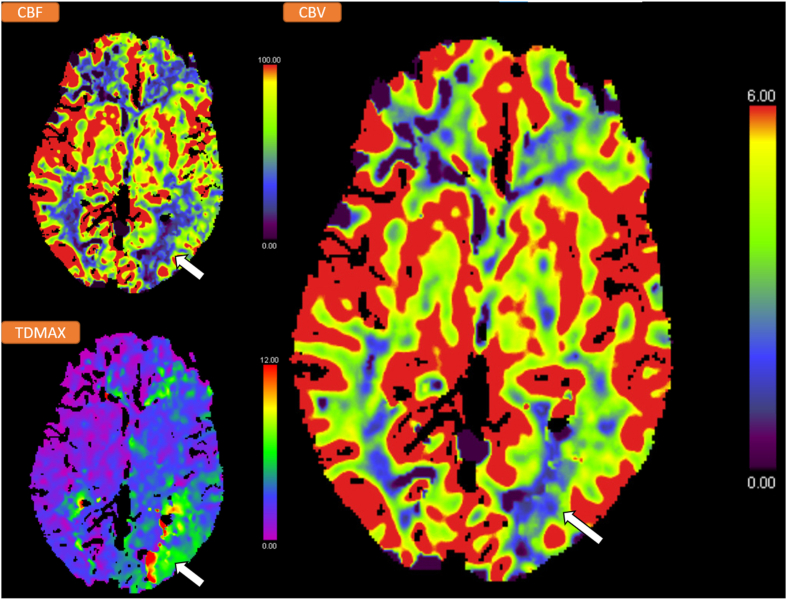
Axial computed tomography (CT) scan of the brain with perfusion images revealing left parieto-occipital increase in the TDMAX and CBF with decrease in the CBV (white arrows) suggestive of small core infarction with surrounding penumbra (brain tissues at risk). CBF: Cerebral blood flow; CBV: Cerebral blood volume; TDMAX: Time to maximum.
